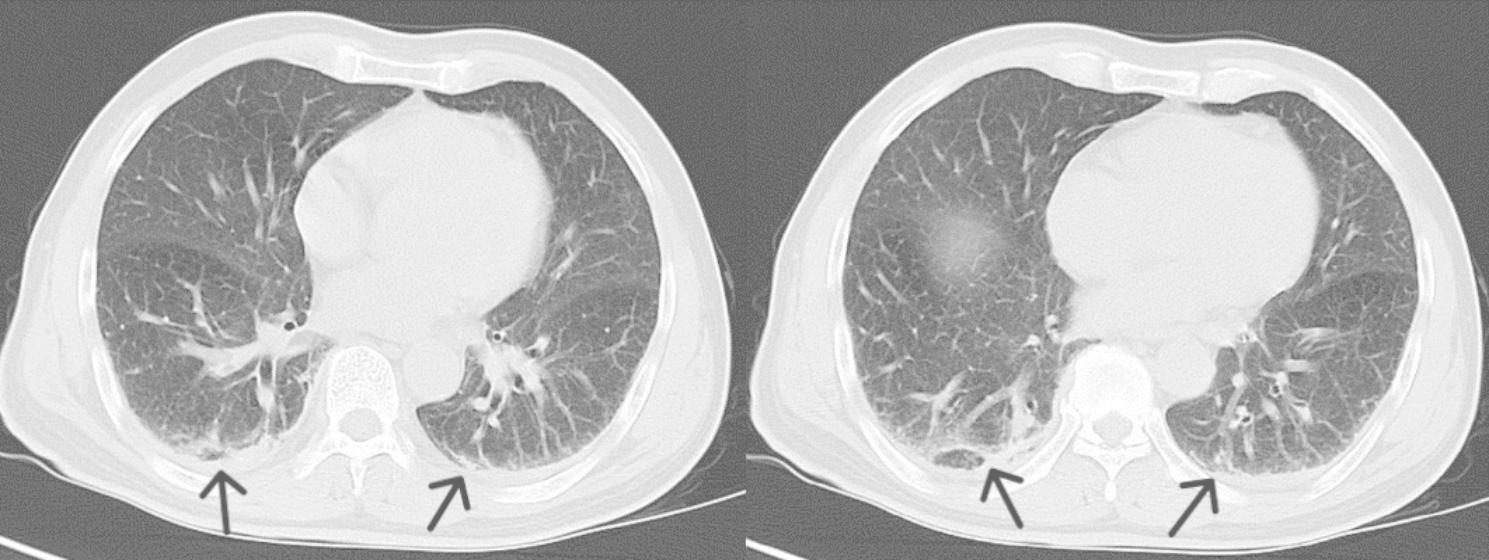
Chest CT of the patient suggesting inflammation of the double lower lungs
